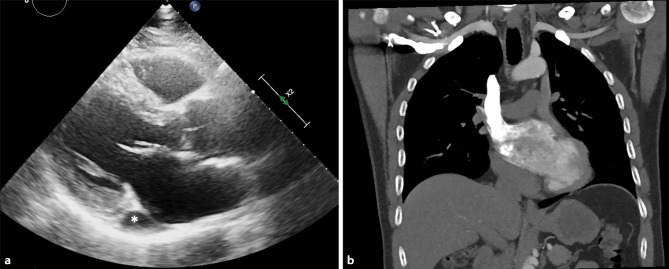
a Transthoracic echocardiography, parasternal long-axis view. The *asterisk* indicates an anomaly. b Computed tomography angiography performed to rule out pulmonary embolism. Contrast was administered via the right arm, coronal plane
